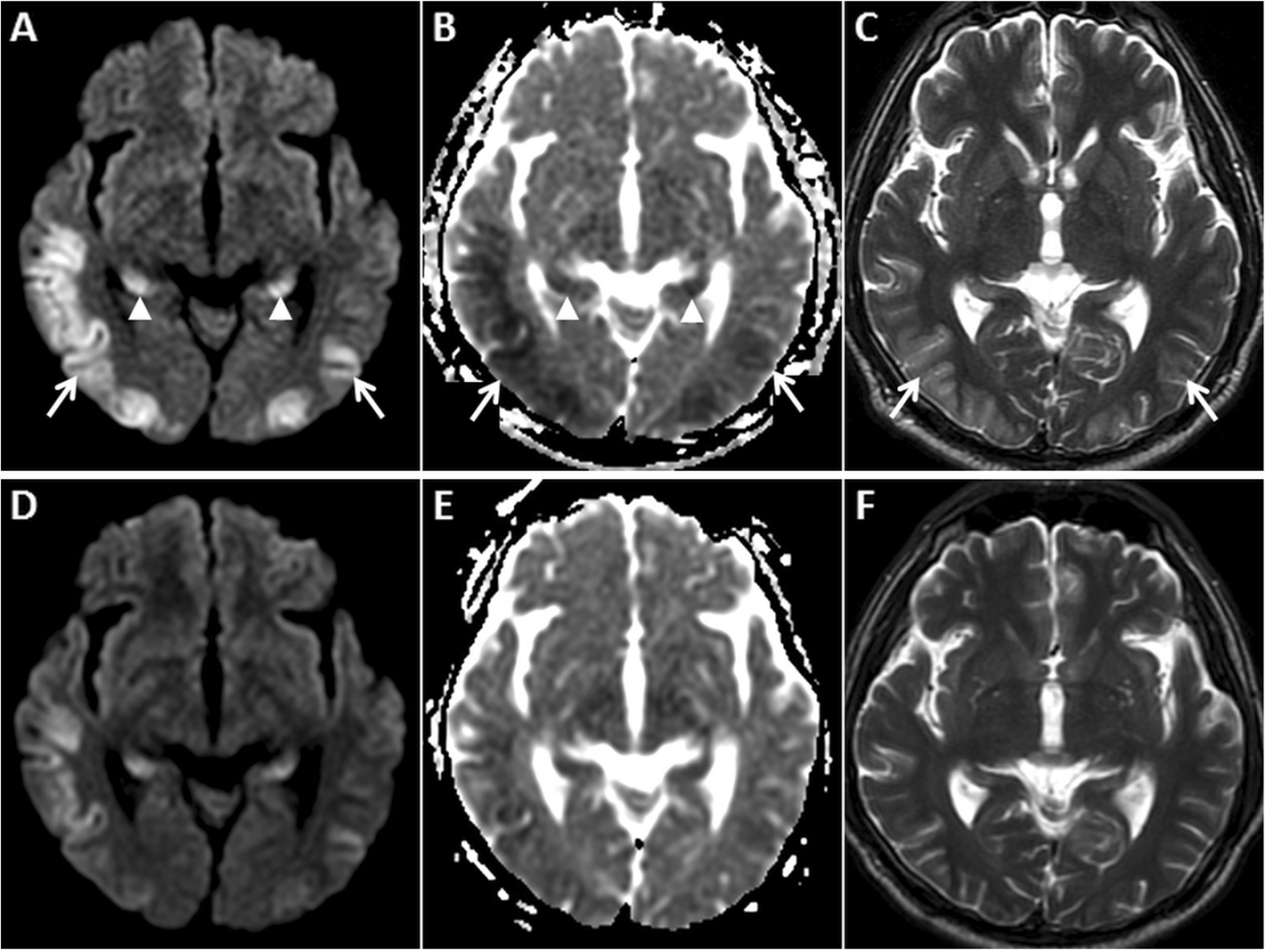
MRI of the brain in a 46-year-old male patient diagnosed with hypoglycaemia on the background of diabetic ketoacidosis. Bilateral asymmetric GRD, more on the right, is seen in the temporo-occipital lobes (arrows) on axial DWI (a) and ADC image (b). Restricted diffusion is also seen in bilateral hippocampi (arrowheads). Mild hyperintensity is noted in the involved cortex (arrows) on the axial T2W image (c). Post-treatment follow-up MRI after 2 weeks shows partial resolution of signal changes on axial DWI and ADC images (d, e, respectively). Note that the axial T2W image also appears almost unremarkable (f)
